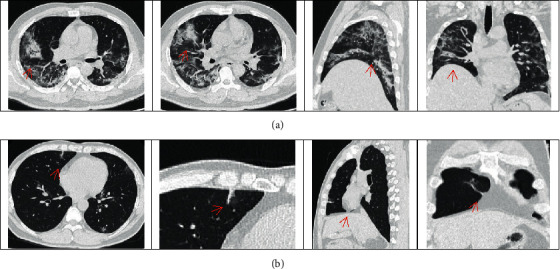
Chest CT findings in COVID-19 patients with T2DM and NDM. (a) Male, 49 years old, common type COVID-19, 10-year history of T2DM. Patchy shadows can be seen in multiple lobes of both lungs, local consolidation can be seen in the middle lobe of the right lung, and air bronchi signs can be seen inside. (b) Male, 41 years old, common type COVID-19, NDM. Patchy shadows are seen in multiple lobes of both lungs, with local consolidation in the middle lobe of the right lung.
